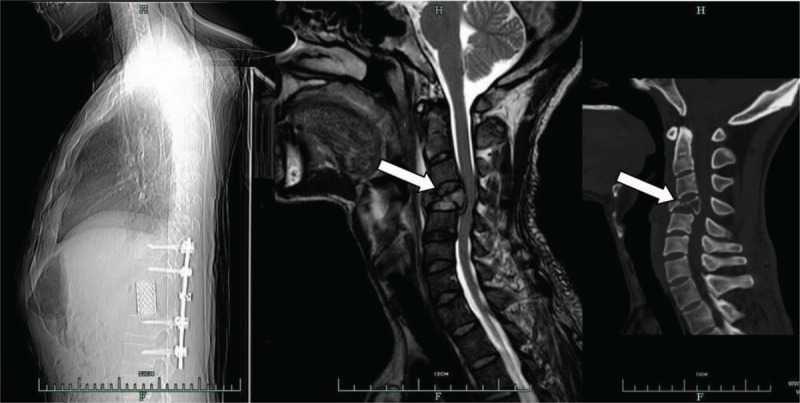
(A) Lateral view of radiographs after total en bloc lumpectomy for T12 metastasis. (B) Sagittal views of the T2-weighted magnetic resonance images and CT images at 3 months post-surgery. Arrows indicate the newly developed metastasis at C4.
